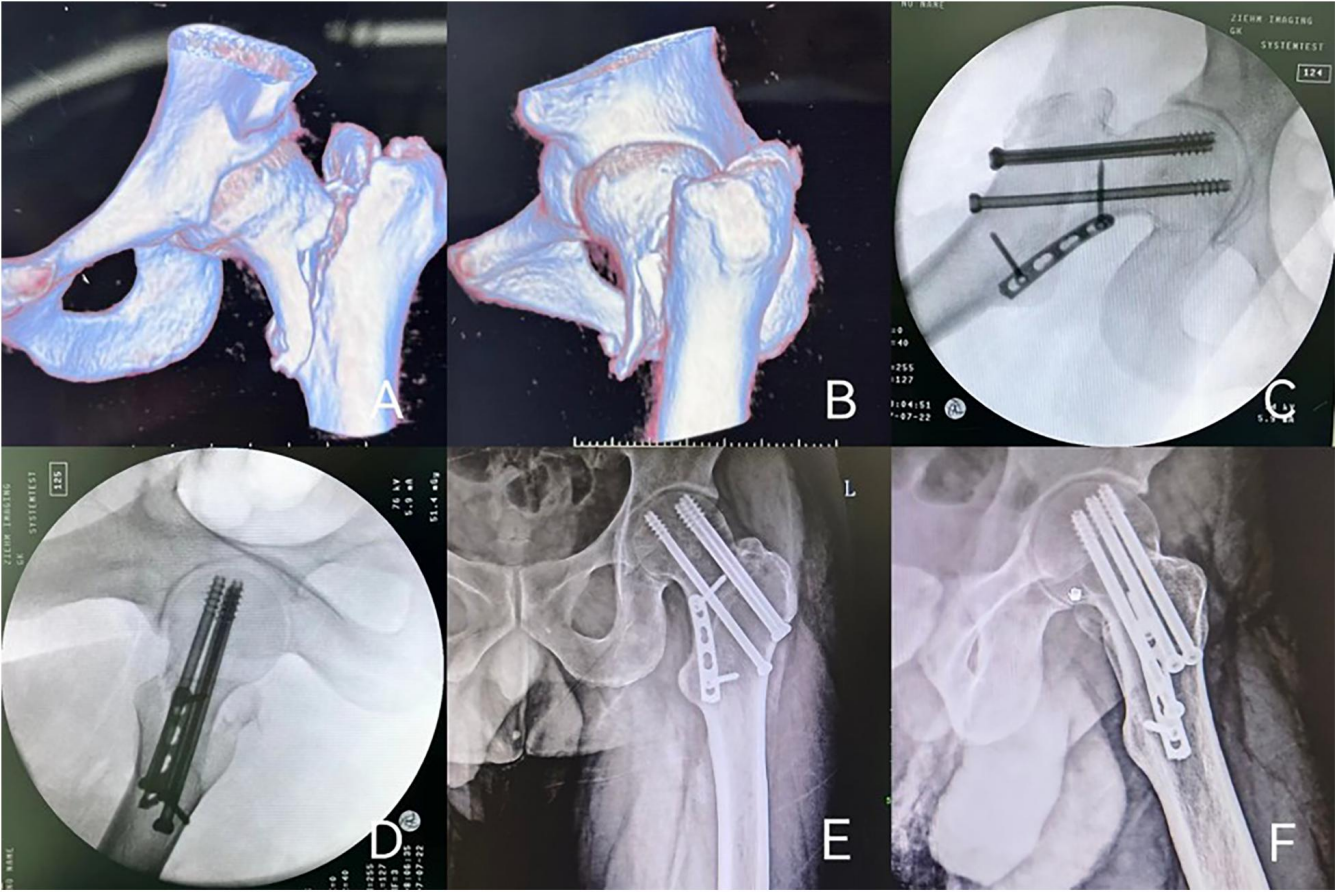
A 45-year-old female patient with left reverse obliquity femoral neck fracture who was treated by cannulated screw (CS), medial buttress plate (MBP). (A,B) Preoperative CT scan suggested a fracture of the left femoral neck (Pauwels III-type, Pauwels angle was 90°); (C,D) the patient was treated with CS, MBP. Excellent reduction was achieved; (E,F) the x-rays examination after 8 months postoperative follow-up showed fracture healing, no femoral neck shortening, and no implant failure.
